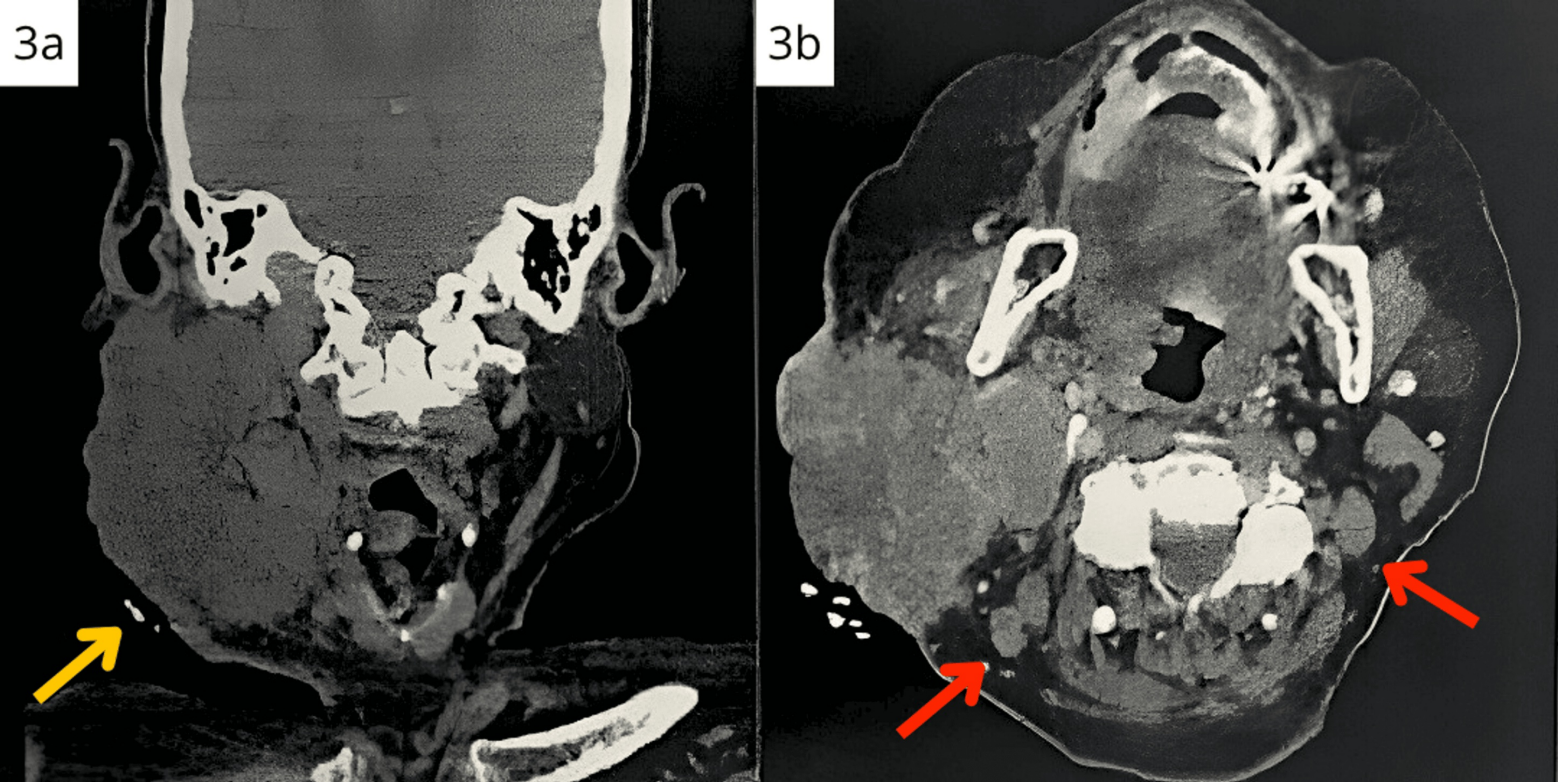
(a) CT coronal image (yellow arrow). Large ill-defined solid tumor in the right neck (9 × 7 × 6.5 cm) with cystic/necrotic components and intralesional vessels. The mass invades the skin, parotid and submandibular glands, masticator and paraspinal muscles, and the retromandibular trigone. It compresses the right internal jugular vein. Infiltrative ipsilateral parotid and right supraclavicular lymphadenopathy are present. No cortical bone destruction or osseous invasion is identified despite extensive soft tissue infiltration of adjacent muscular structures. (b) CT transversal image (red arrow). Infiltrative lymph nodes, up to 20 mm, are observed in the peritumoral and residual ipsilateral parotid gland regions (approximate maximum clinical diameter: 10 cm).
